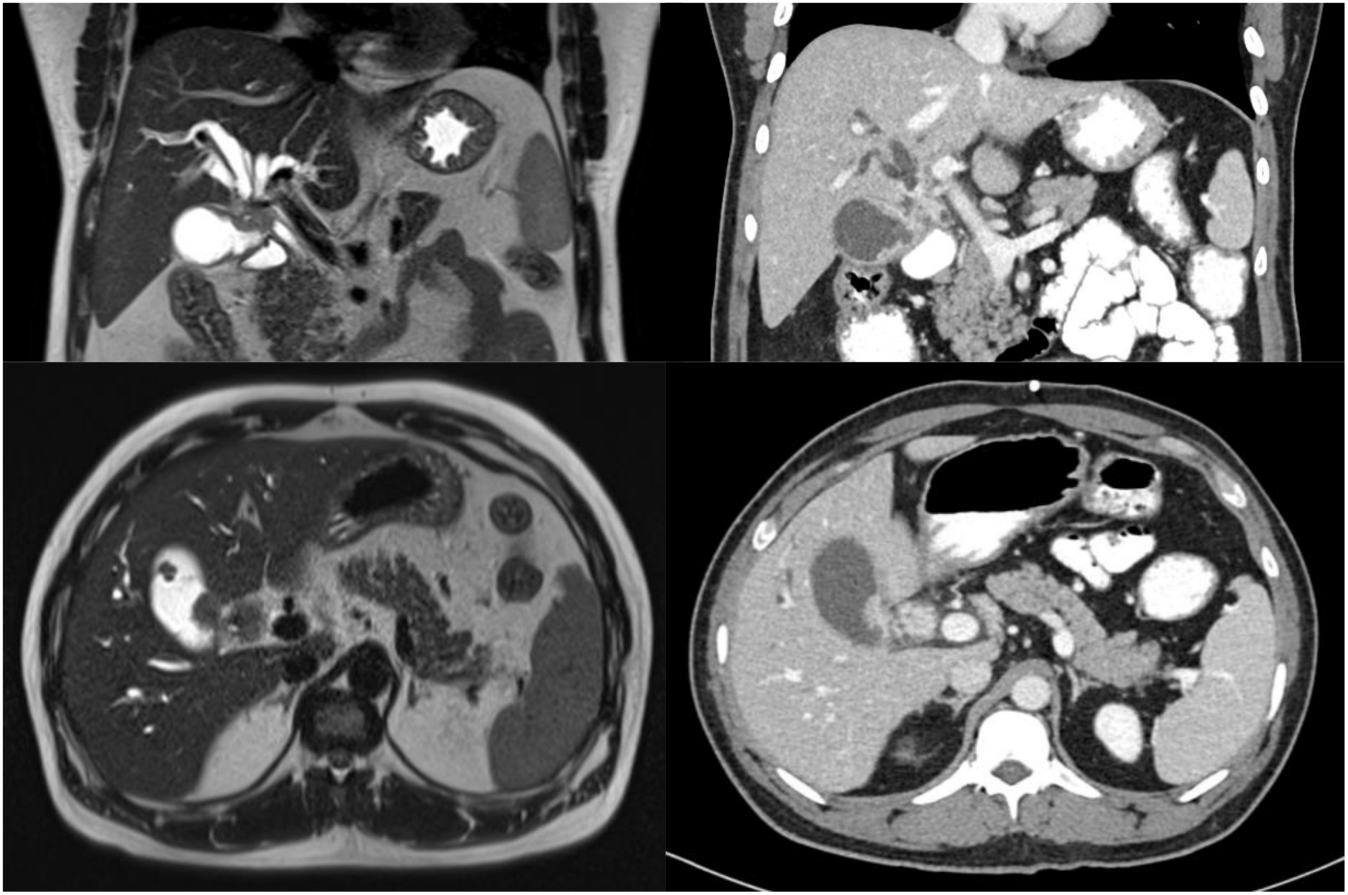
Initial MRI (left) and CT (right) scans demonstrating soft tissue mass involving the common hepatic duct bifurcation, cystic duct and gallbladder.
